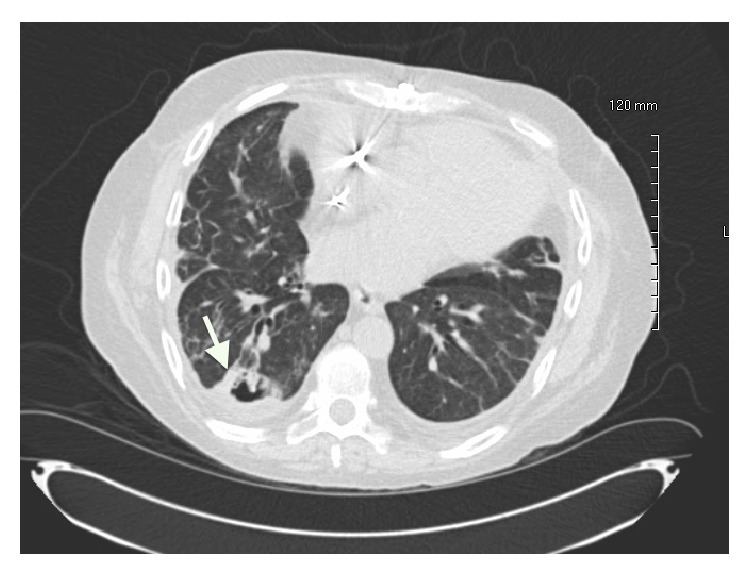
Axial chest computed tomography scan after two weeks of antifungal therapy showing thick-walled, subpleural cavitation and nodules (arrow).
